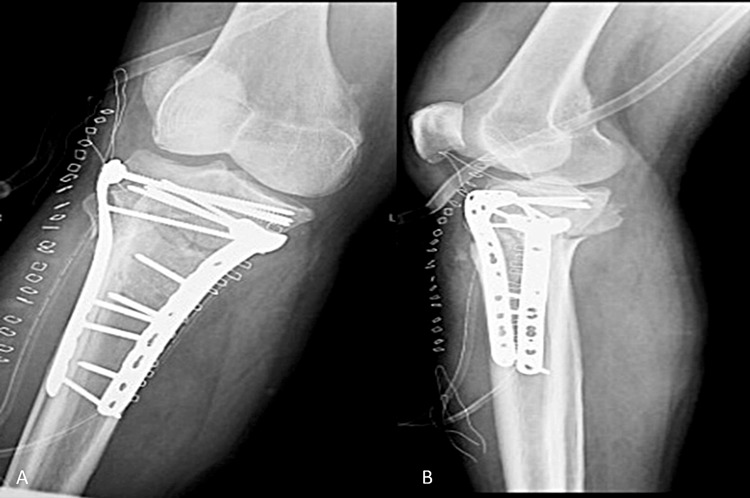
Postoperative radiographs of the right knee joint. (A) Anteroposterior and (B) lateral radiographs show the restoration of the articular surface and of the mechanical axis by open reduction and internal fixation.
